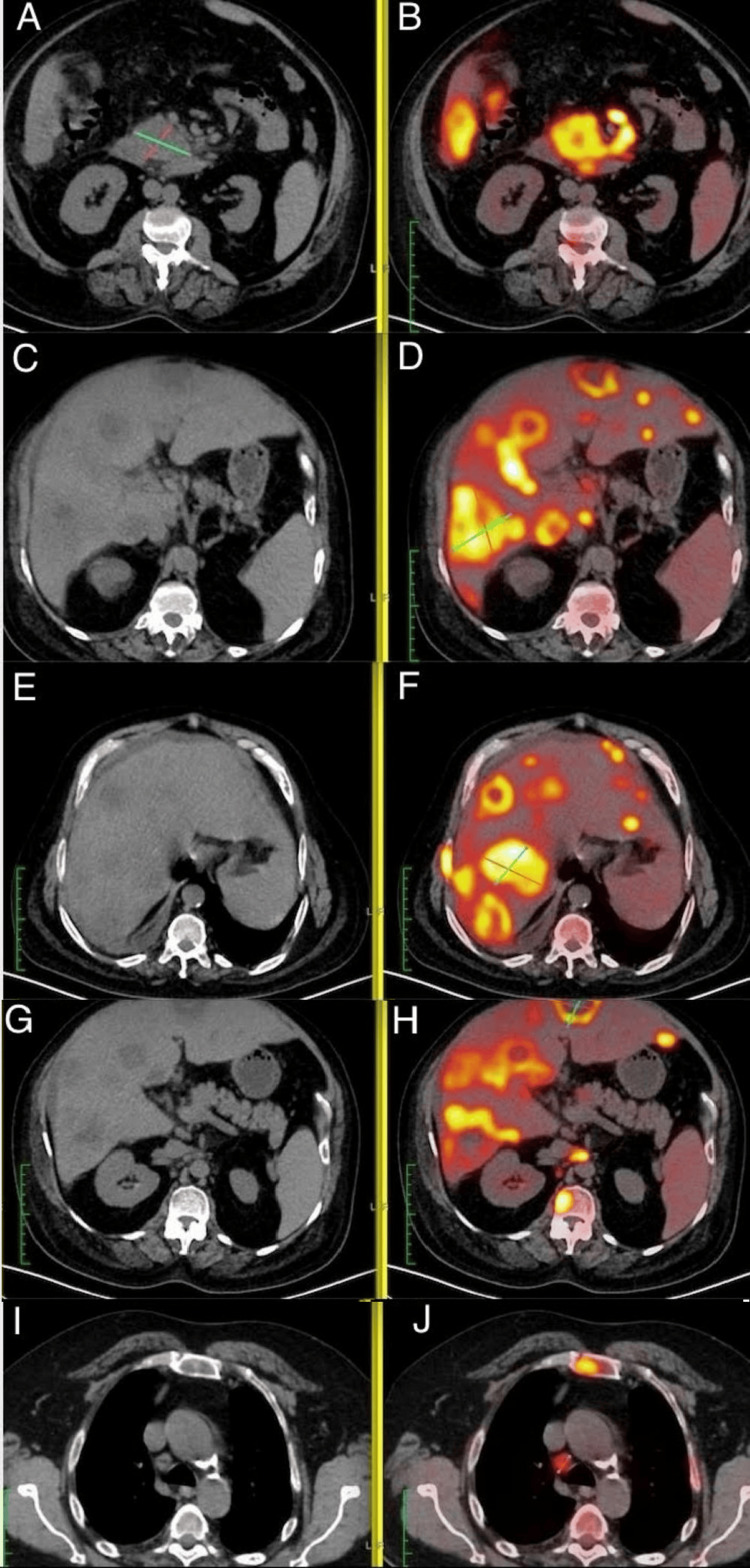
PET-CT demonstrating an FDG-avid lesion in the pancreatic uncinate process (A,B), multiple hypermetabolic hepatic lesions (C-H), and focal skeletal uptake (I,J), consistent with widespread metastatic disease PET-CT: positron-emission tomography computed tomography; FDG: fluorodeoxyglucose
